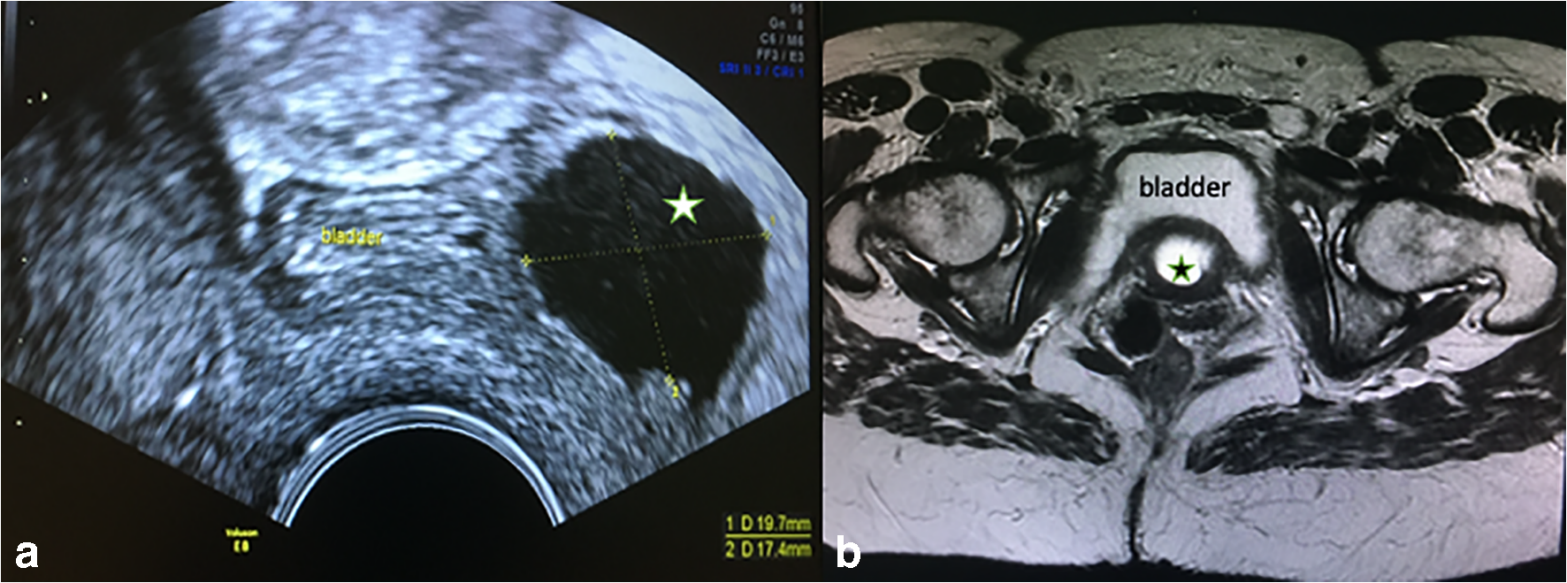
Magnetic resonance image at the level of the bladder above the urethra. The* star* demonstrates the fluid of the diverticulum
